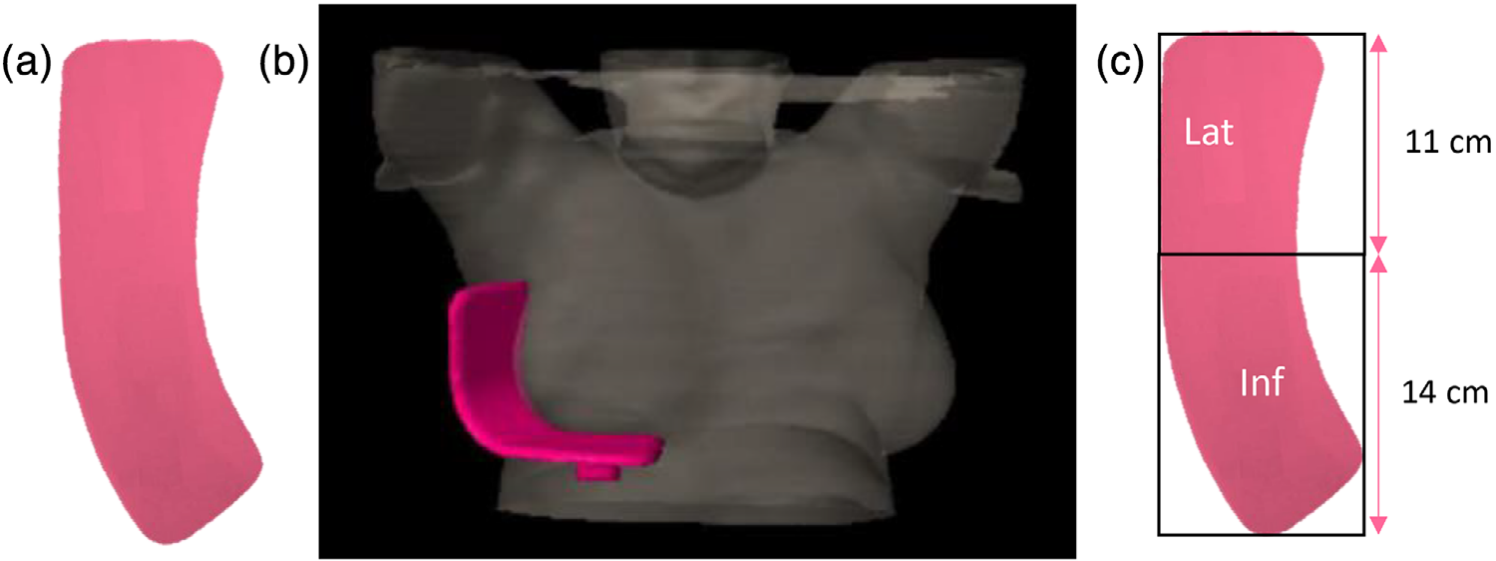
(a) Film cut from stencil to fit surface of breast cradle, flattened into 2D. (b) Breast cradle (pink contour) on a 3D reconstructed CT scan of a patient in the study. (c) Region of interest divided into lateral (lat) and inferior (inf) segments.
